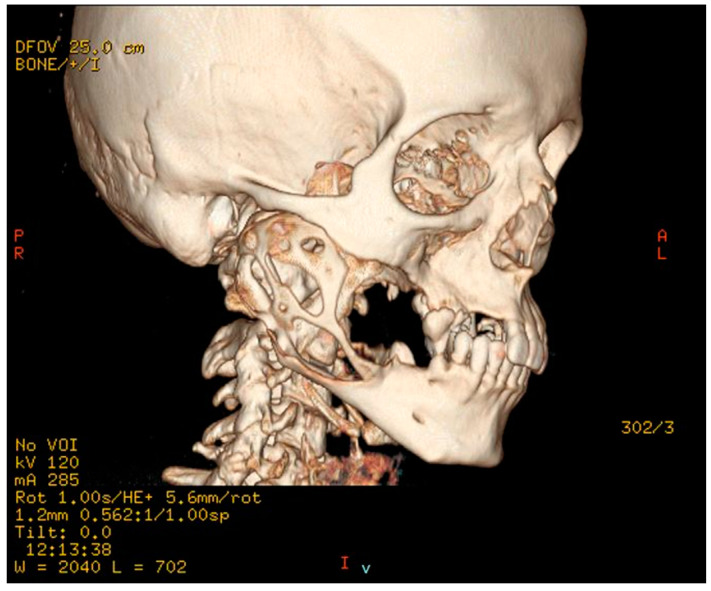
CT scan 3D reconstruction showing the huge lesion extending from dental elements and 5 to the entire right mandible ramus involving the right mandibular body and the alveolar nerve canal, with perforations of the cortical bone wall on both buccal and lingual sides.
